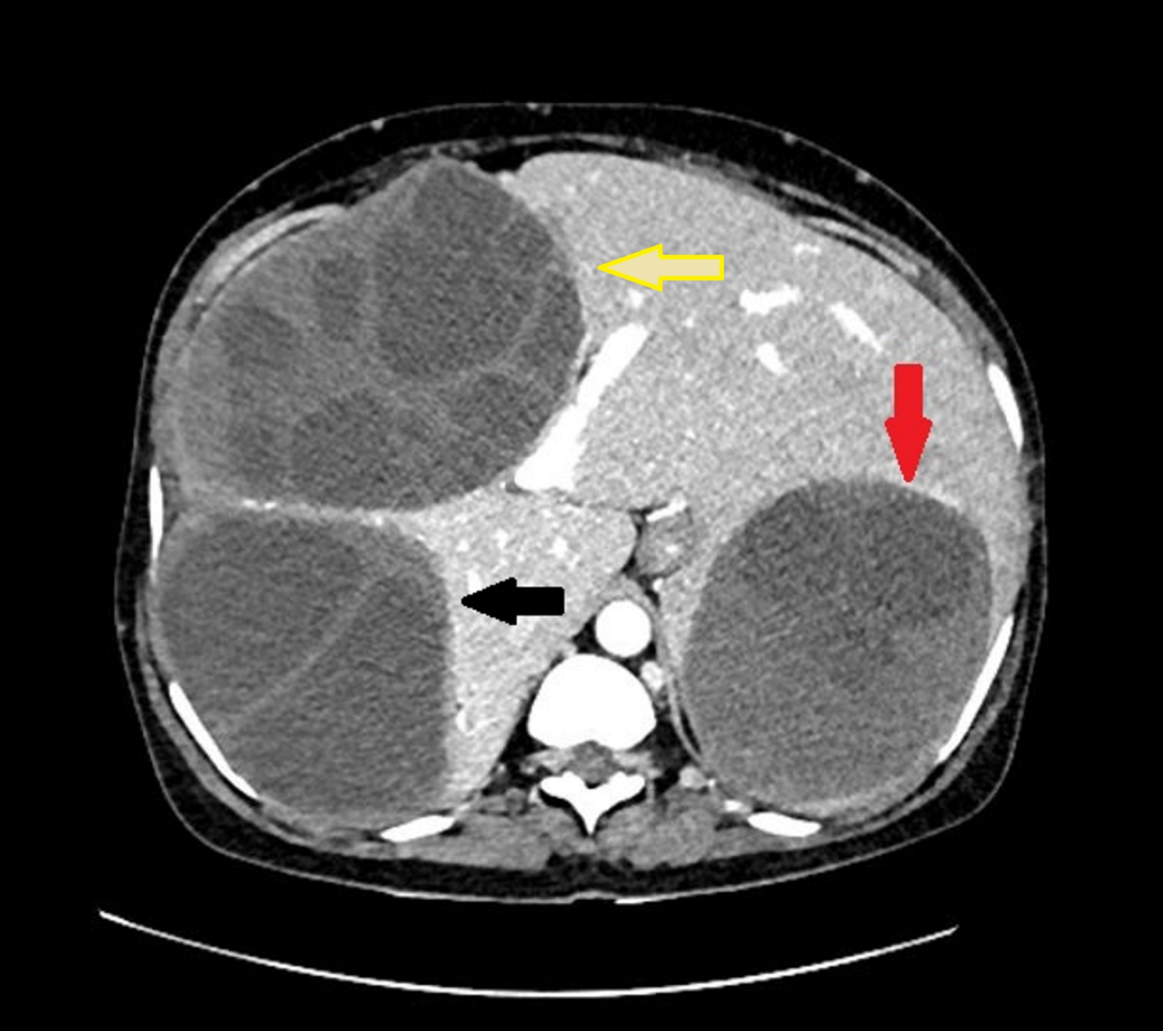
Hydatid cyst in the liver and peritoneum on CT CECT abdomen axial image showing a large, well-defined hypodense lesion in the right lobe of the liver (black arrow) with mildly enhancing wall and internal septations. Similar hypodense lesion is seen in the left lobe of the liver (red arrow) with debris within and no septations. Another moderate-sized, well-defined, rounded cystic lesion with mildly enhancing walls and internal septations is seen in the peritoneum (yellow arrow) with scalloping of the adjacent liver margins. CECT: contrast-enhanced computed tomography, CT: computed tomography
